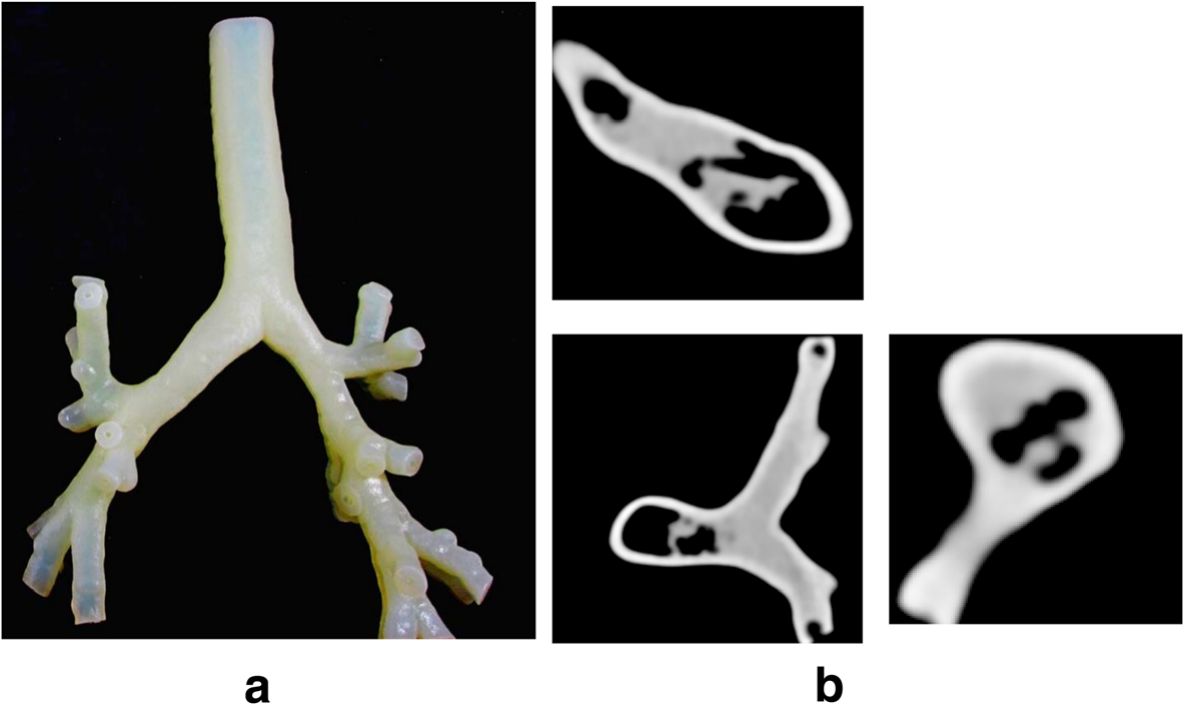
CT scan of a trachea model (a) showed residual supporting materials inside (b)
